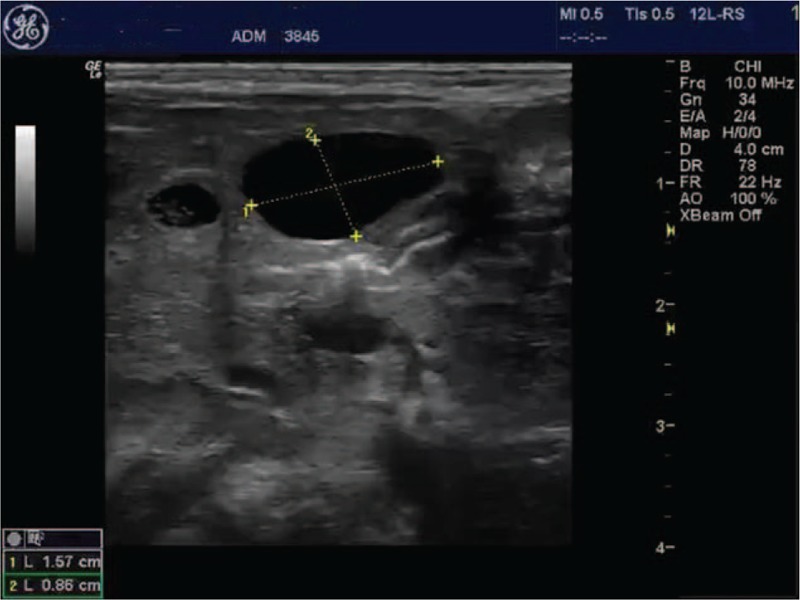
Transabdominal ultrasound immediately after delivery (using a linear probe).
